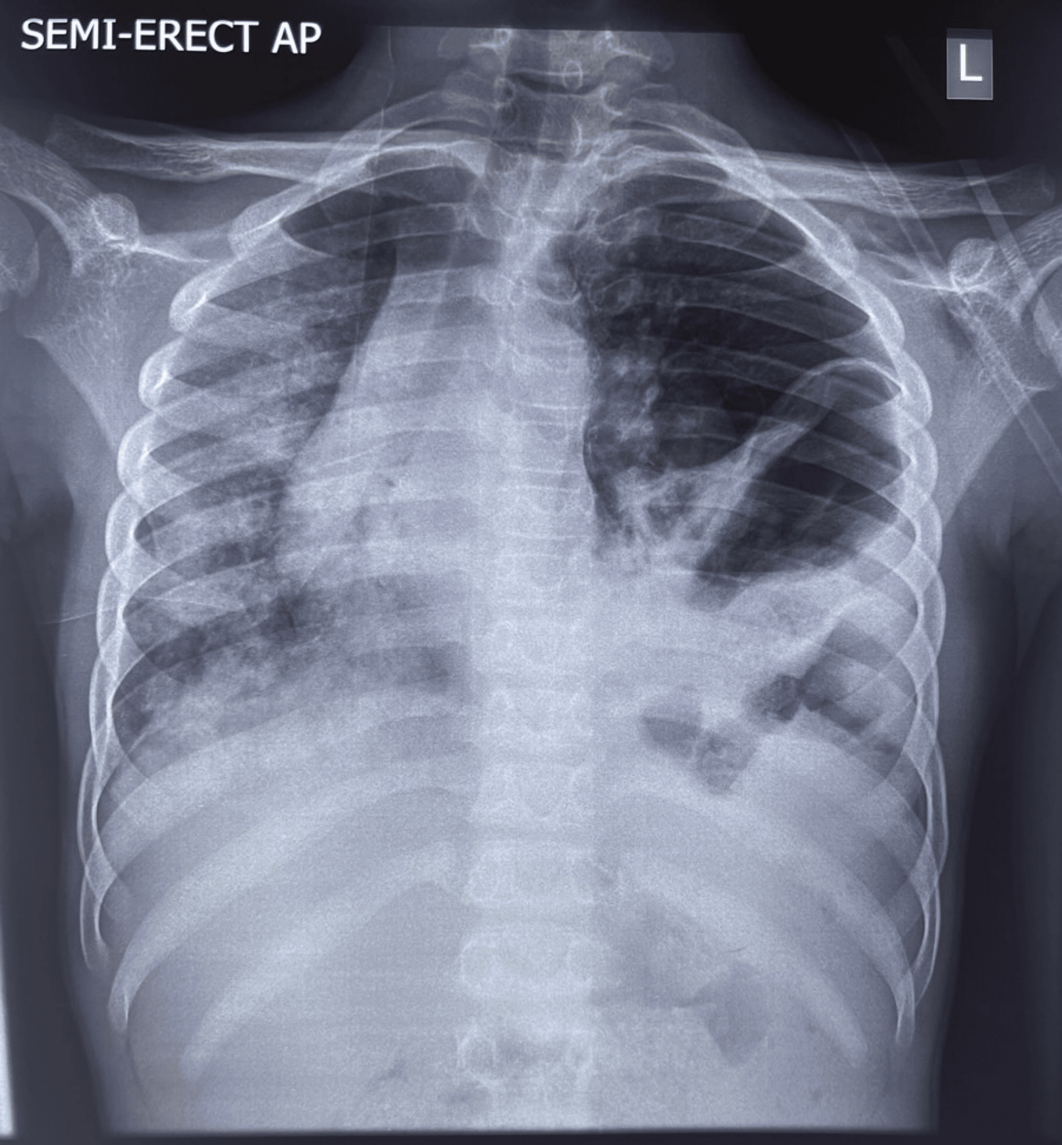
Semi-erect chest X-ray showing rightward shift of the trachea and mediastinum, absent lung markings on the left side, and a disrupted left hemidiaphragm
